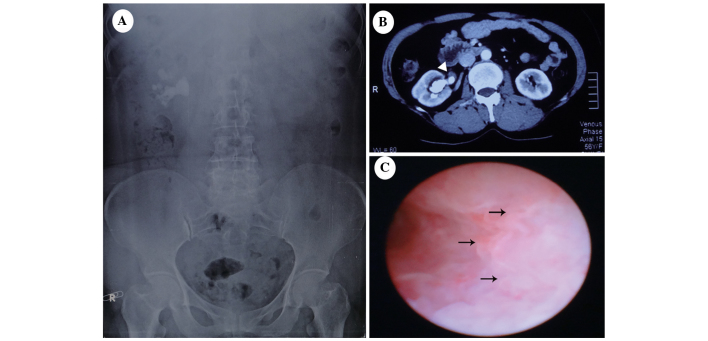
Imaging diagnosis of the patient. (A) Abdominal X-ray revealed right renal staghorn calculi. (B) Contrast enhanced computed tomography demonstrated slight contrast enhancement in the right renal pelvis (white triangle). (C) Mucosal changes under ureteroscopy in the renal pelvis (black arrows).
